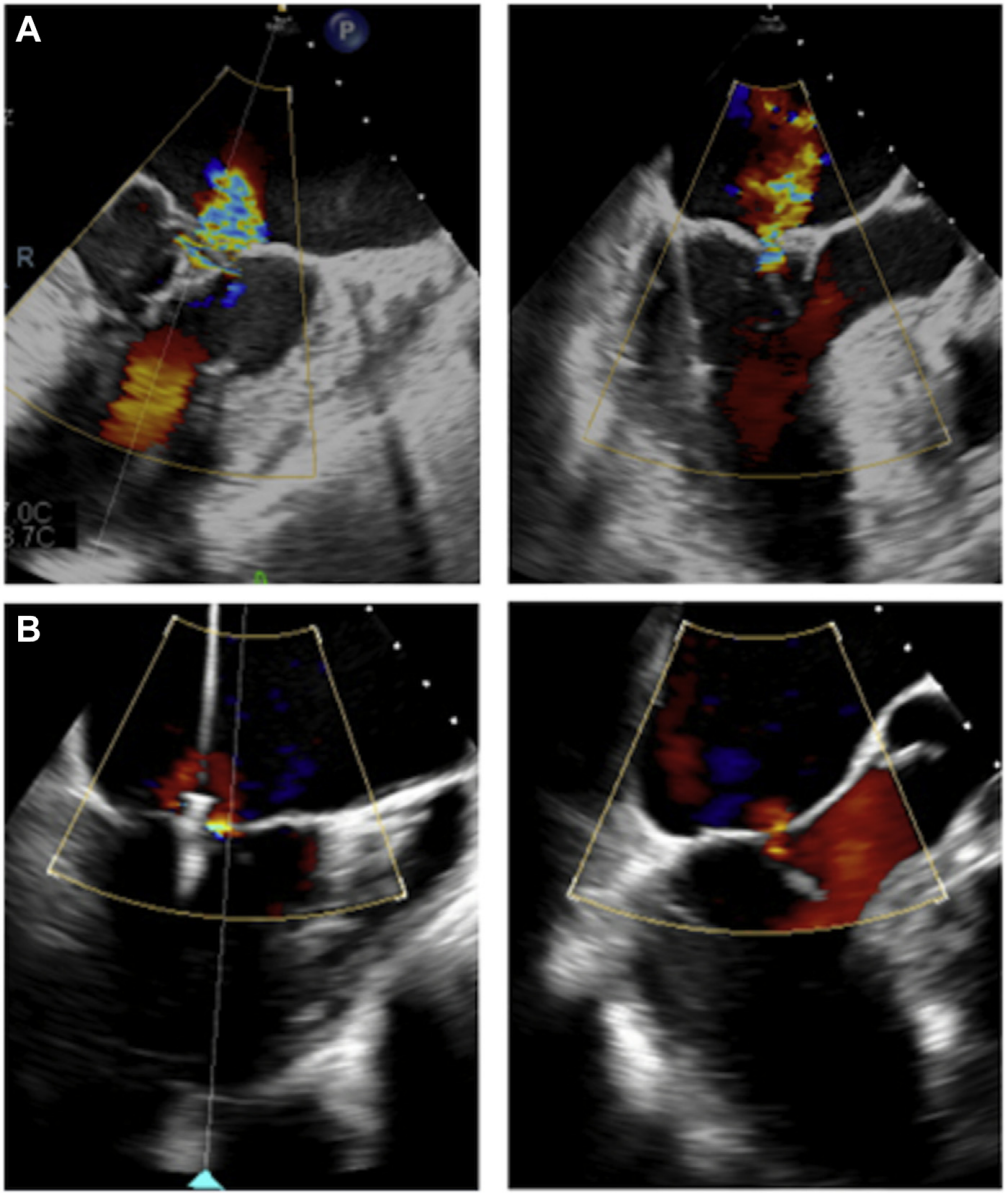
Transesophageal echocardiography X-plane views during MitraClip (Abbott Laboratories, Abbott Park, IL) procedure showing intermediate functional mitral regurgitation under general anesthesia (A). First clip installed in zone 2, leaving a residual eccentric lateral mitral regurgitation caused by a partial systolic anterior motion (B).
